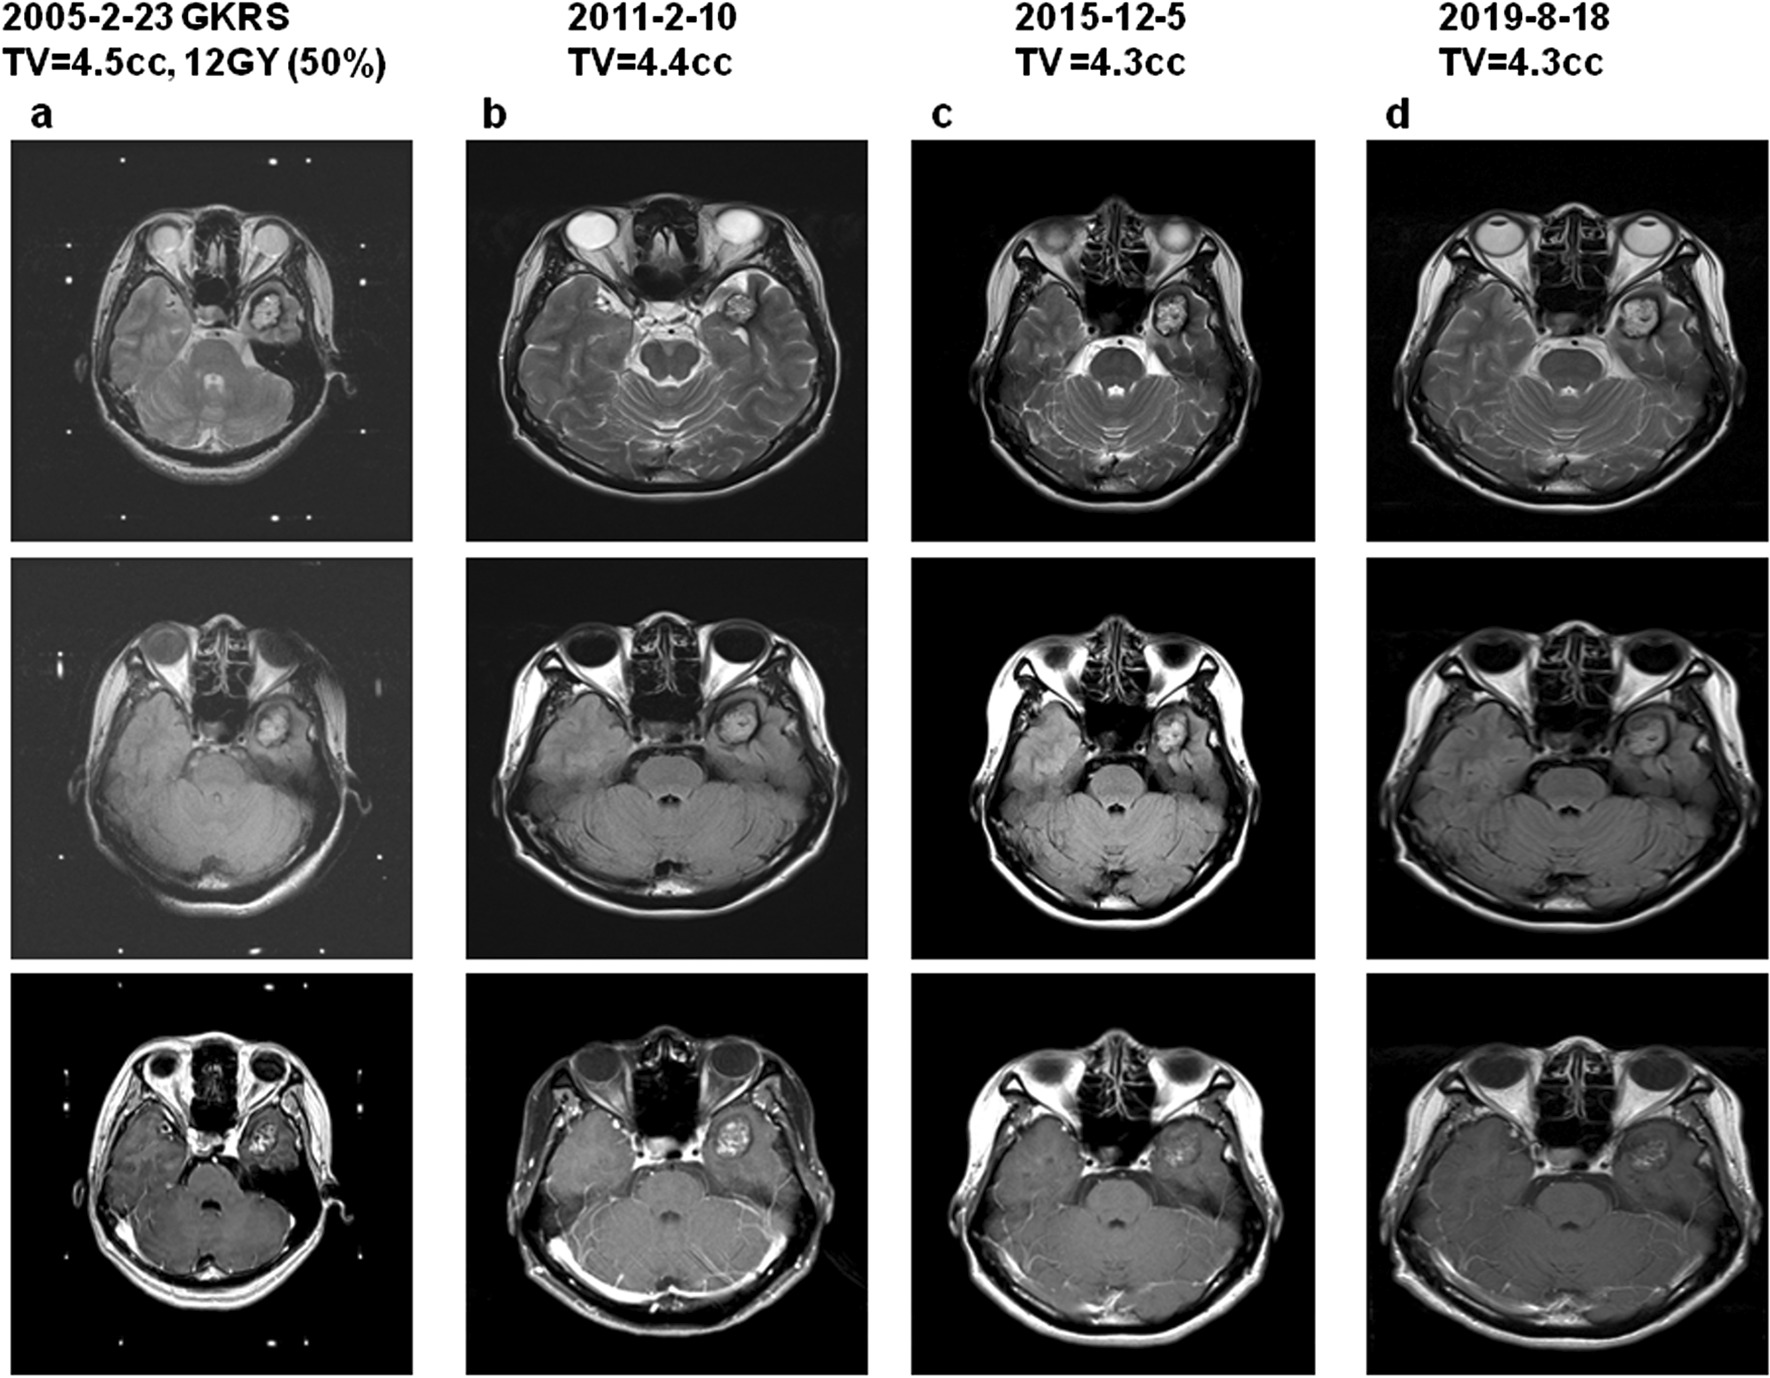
Cavernoma treated with GKRS and achieving stable size. A 30 year old female presented with tonic–clonic seizure treated with Gamma Knife radiosurgery with stable size of nidus and seizure control of Engel II. a MRI imaging of T2, Flair and T1 with contrast at the time of GKRS with radiation volume of 4.5 cc with 12 Gy in 50% line. b MRI imaging of T2, Flair and T1 with contrast 6 years after GKRS with nidus volume of 4.4 cc, c MRI imaging of T2, Flair and T1 with contrast 10 years after gamma knife treatment with nidus volume of 4.3 cc and d MRI imaging of T2, Flair and T1 with contrast 14 years after GKRS with nidus volume of 4.3 cc
